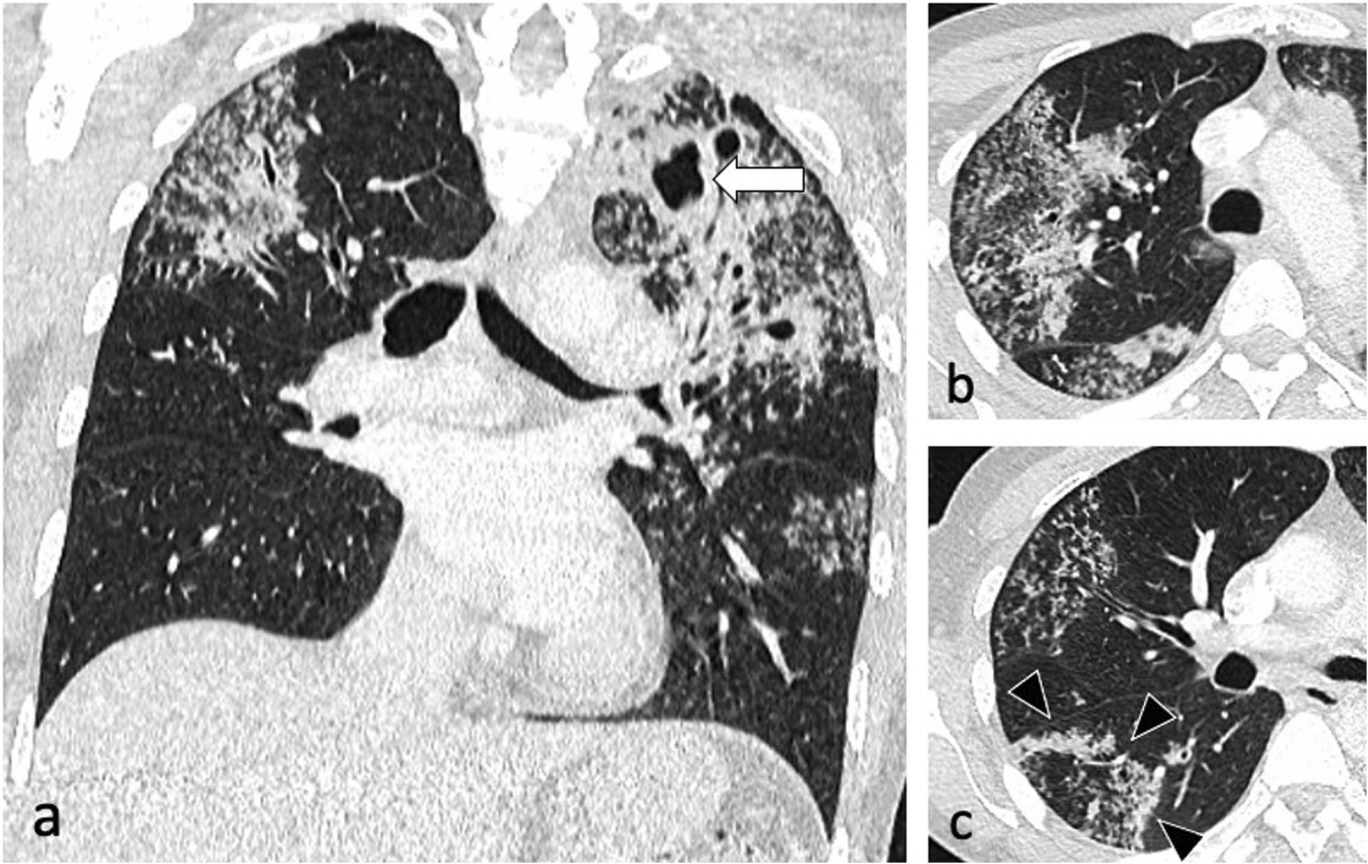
A 47-year-old female with a known diagnosis of HIV presented with weight loss, night sweats and worsening dyspnoea. Her CD4 count at presentation was 120 cells/mm^3^. CT (lung window) demonstrates thick-walled irregular cavities (coronal plane—a, arrow), extensive bilateral upper lobe centrilobular and tree-in-bud nodules and nodular reversed halo sign in the apical segment of the right lower lobe (axial plane—c, arrowhead)
